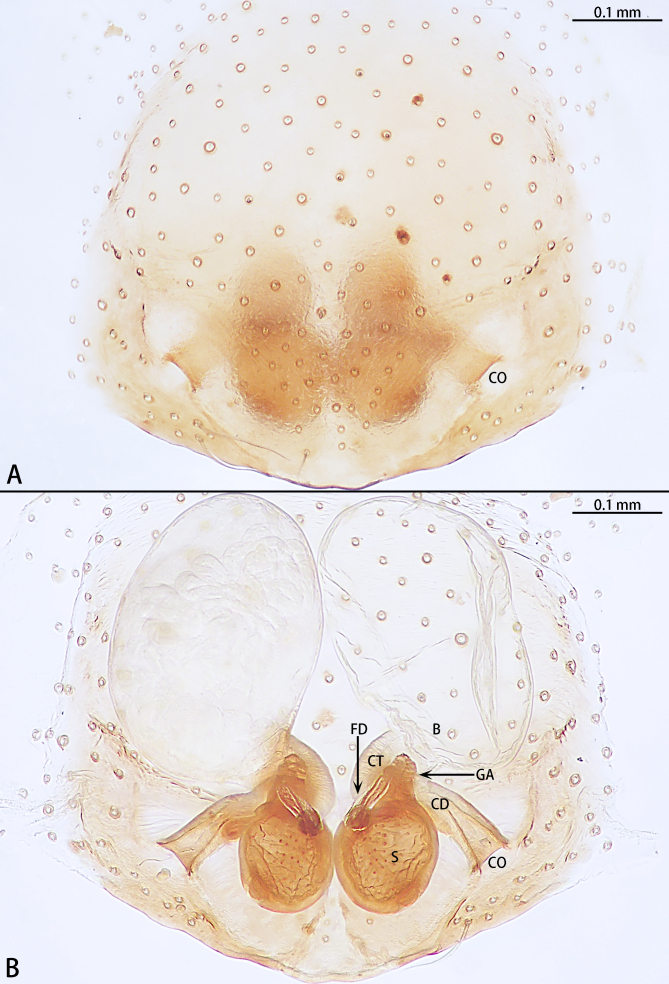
Otaciliakhezu* sp. nov., paratype female. A Epigyne, ventral view; B Vulva, dorsal view. Abbreviations: B bursa; CD copulatory duct; CO copulatory opening; CT connecting tube; FD fertilisation duct; GA glandular appendage; S spermathecae.
